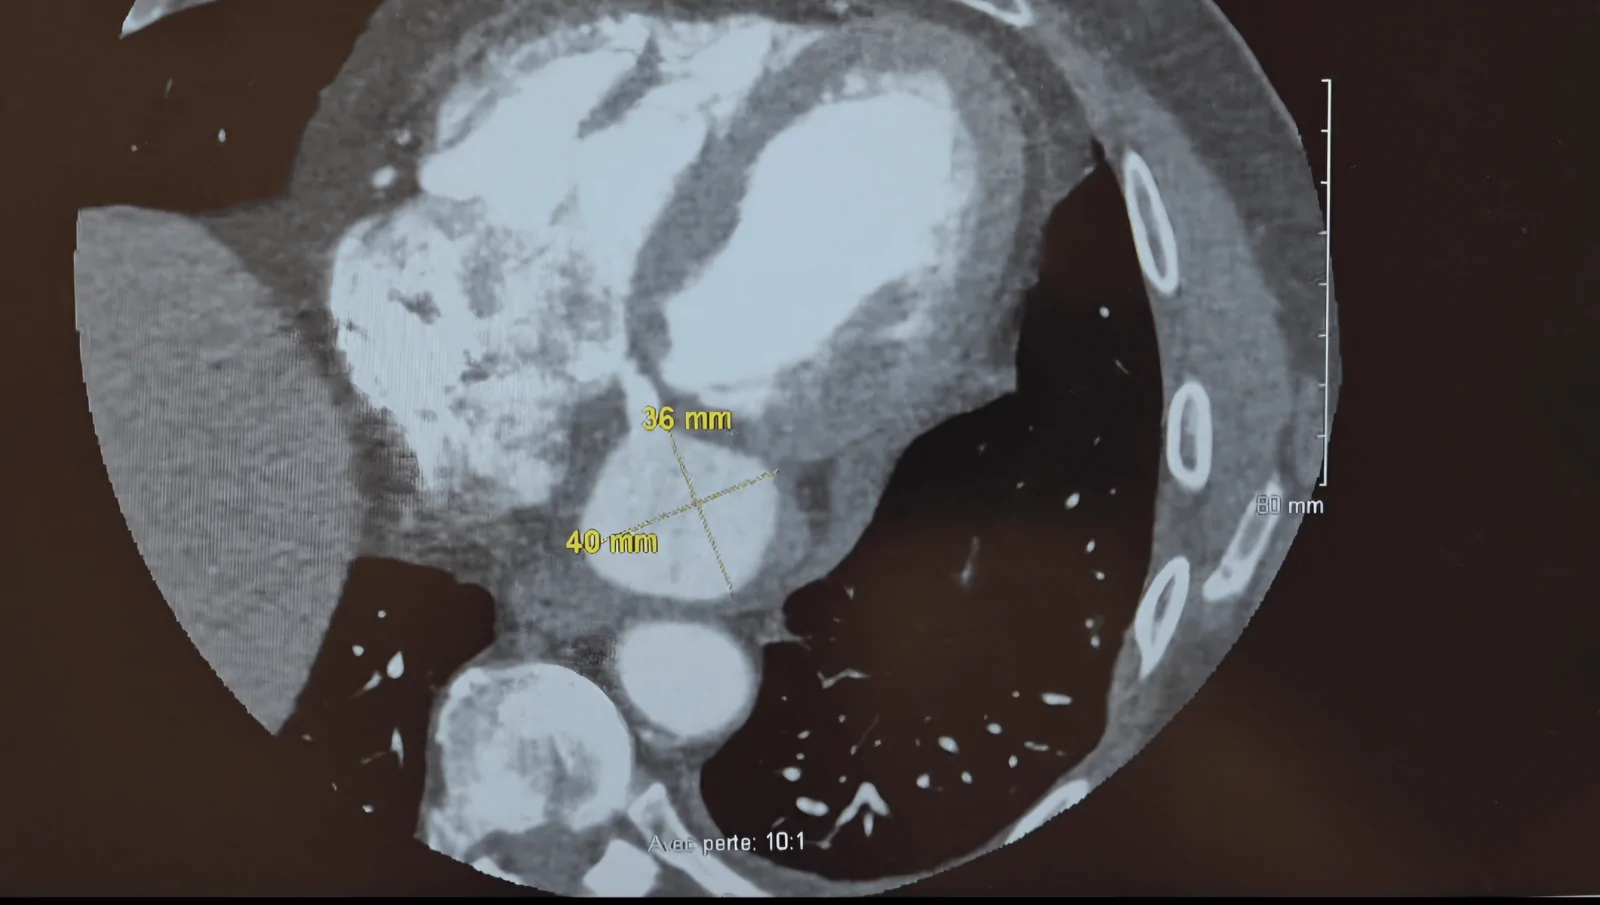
Contrast-enhanced cardiac computed tomography (CECT) performed three months after mitral valve replacement The image is demonstrating a persistent, stable left atrial pseudoaneurysm measuring 40 × 36 mm adjacent to the posterior wall of the left atrium.
